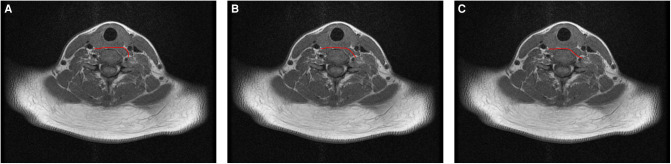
Prespinal routes of CC7 transfer surgery on T1-weighted images (T1WI): (A) anterior route, (B) middle route, (C) posterior route.
